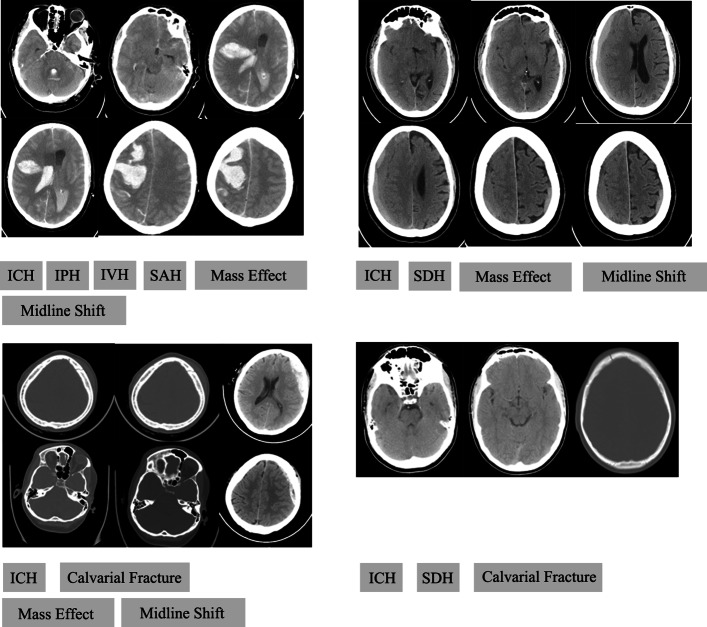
Example CT images from the dataset. We can see that a full-slice brain CT scan can reflect multiple diseases, and for an accurate diagnosis of the disease, multiple images are required. Therefore, we cannot only treat a single slice image. Learning the dependencies of the full-slice is very important. (ICH: Intracranial haemorrhage, IPH: Intraparenchymal, IVH: Intraventricular, SAH: Subarachnoid, SDH: Subdural)
